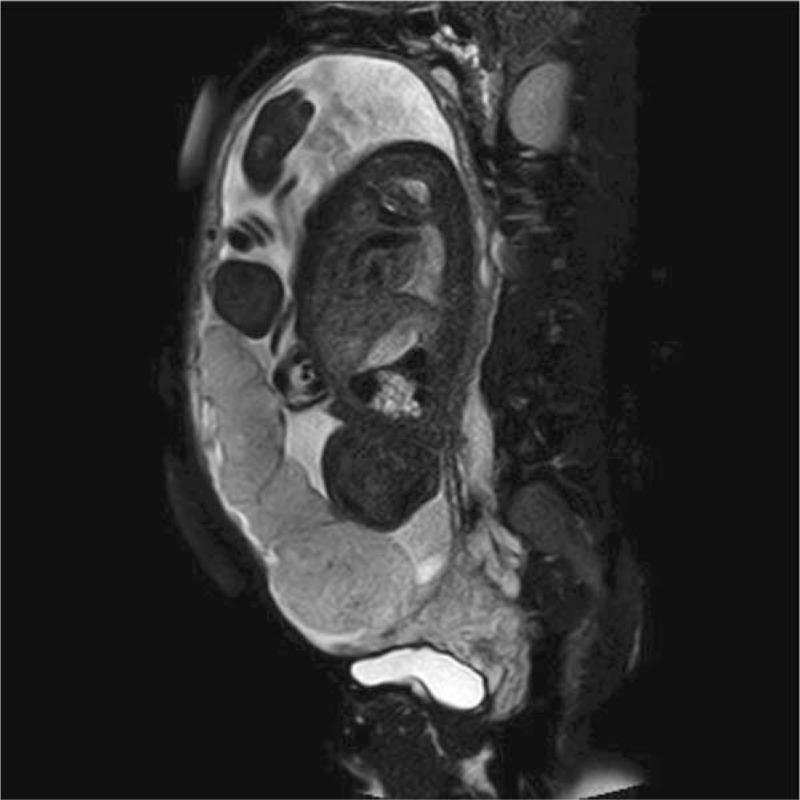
Magnetic resonance imaging (MRI) before cesarean section. It showed the pernicious placenta previa and placenta accreta in the anterior inferior uterine incision scar site.
